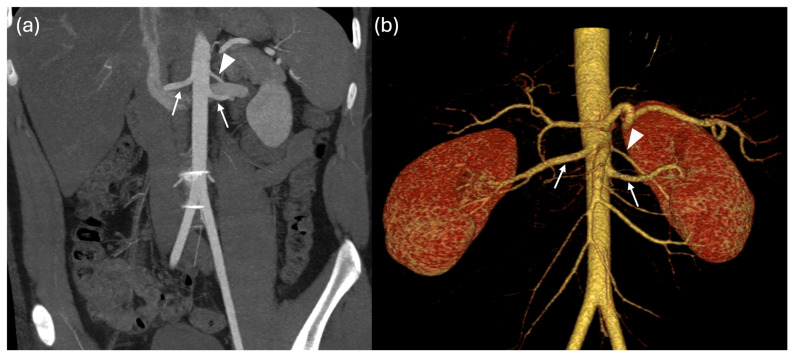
Coronal MIPr (a) and volume rendering (b) CT images show the main renal arteries (arrows) and an accessory artery (arrowhead) directed to the posterior segments of the left kidney.
